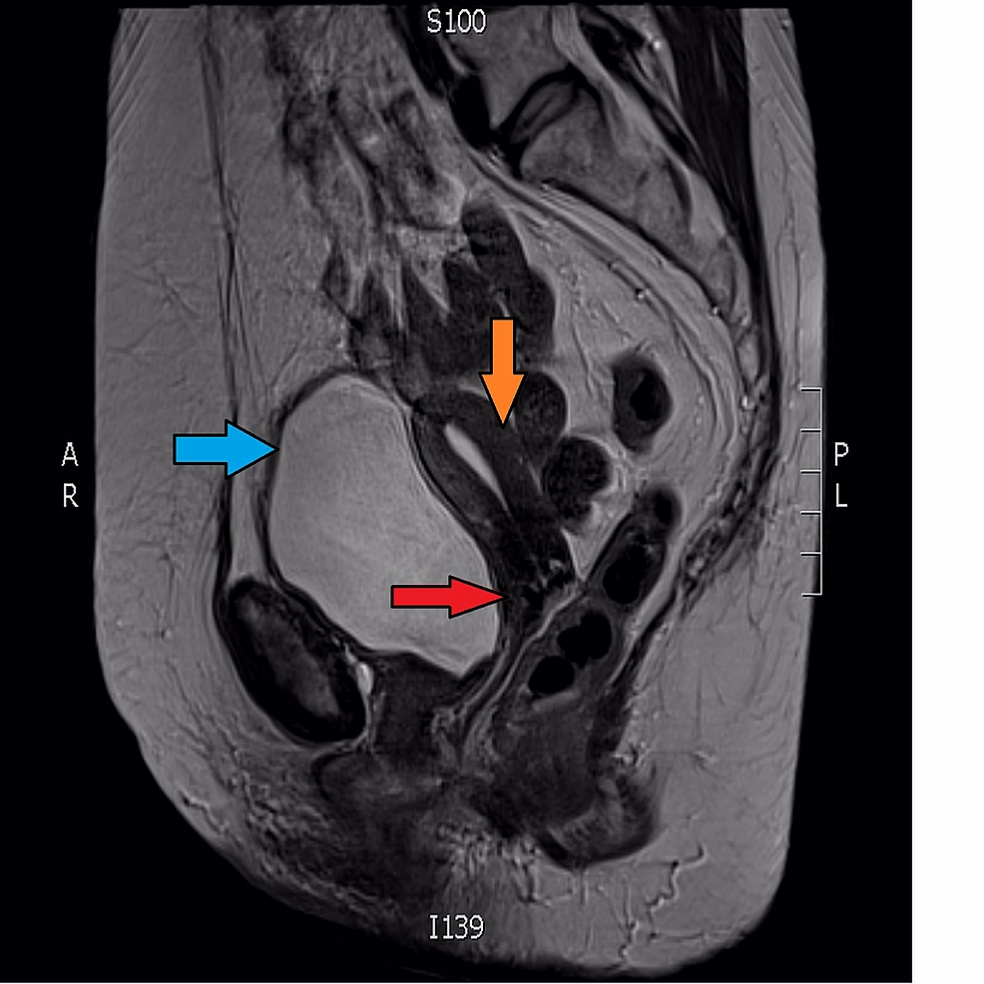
Sagittal view from MRI scan Blue arrow: Bladder; Orange arrow: Fundus of the uterus with a minimal amount of fluid in the endometrial cavity; Red arrow: Cervix
